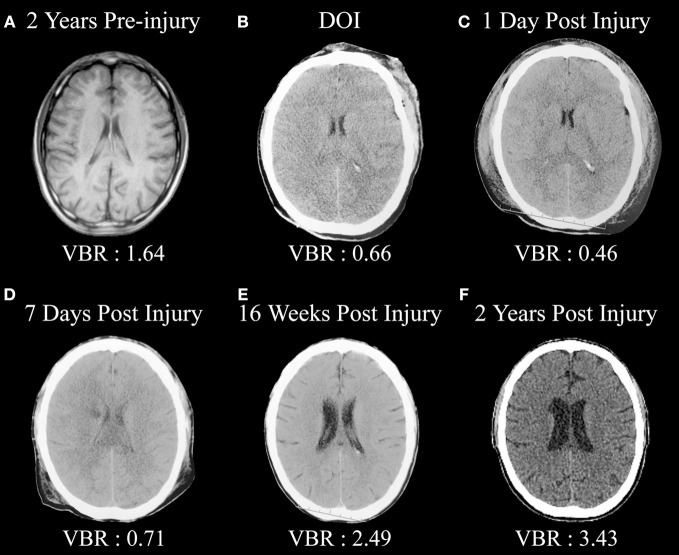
(A) Pre-injury magnetic resonance image (MRI) approximately 2 years prior to a severe traumatic brain injury (TBI). Note the normal size of the ventricular system and ventricle-to-brain (VBR) ratio of 1.64 (normal is approximately 1.5 with a 0.5 standard deviation). (B) Day-of-injury initial CT demonstrating brain edema and reduced VBR, which continues to be reflected in (C,D). (E) Distinct neurodegeneration has occurred by 16 weeks post-injury, reflected as ventricular dilation and increased VBR, with continued neurodegeneration out to 2 years post-injury as seen in (F).
